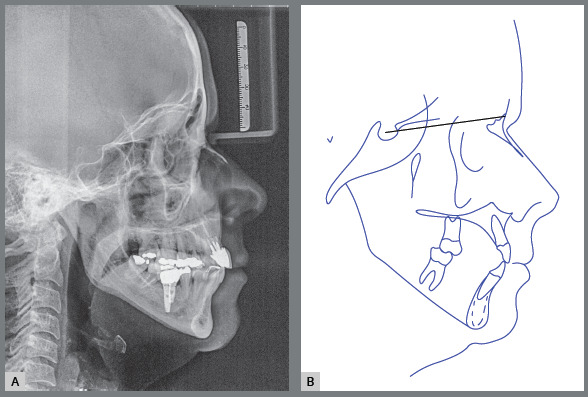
Cephalometric radiograph (A) and cephalometric
